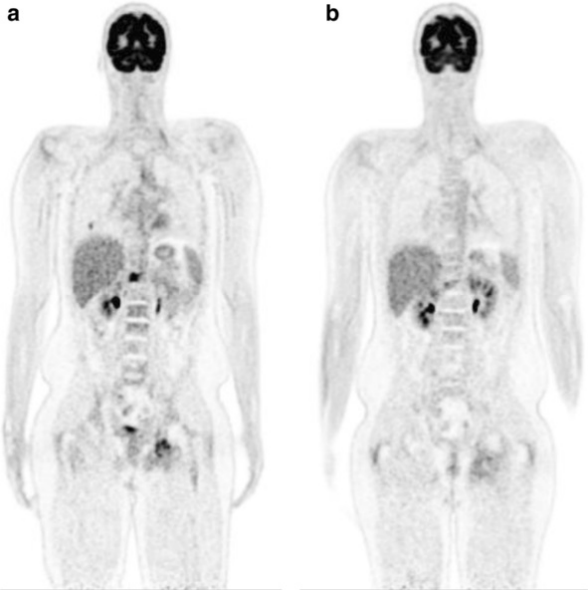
Coronal ^18^F-FDG PET-CT scans done prior to (a) and after (b) 3 cycles of pembrolizumab. The markedly increased FDG uptake in the right side and adjacent soft tissues of T12, in the left ischium and in one of the right middle lobe pulmonary metastases are shown. Post-treatment the FDG avidity in the bony lesions is much reduced and the right middle lobe lesion had completely resolved
